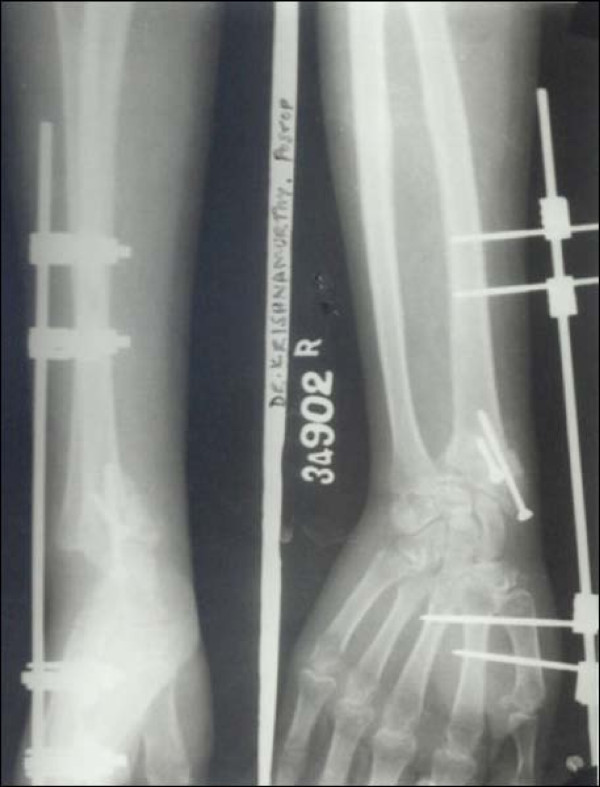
Case 1 Postoperative Radiograph - Anteroposterior and Lateral view.
